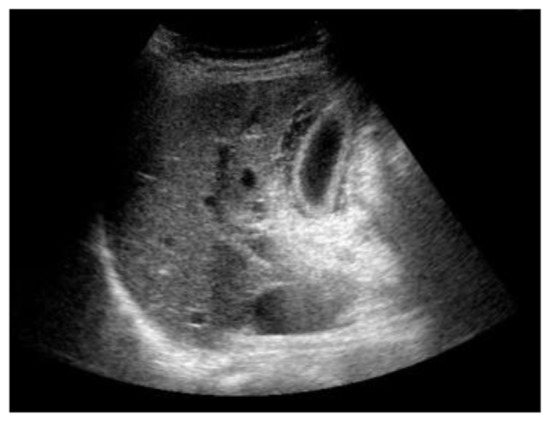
Abdominal ultrasonography revealed thickening of the gallbladder wall without gallbladder stones or emphysematous changes.
